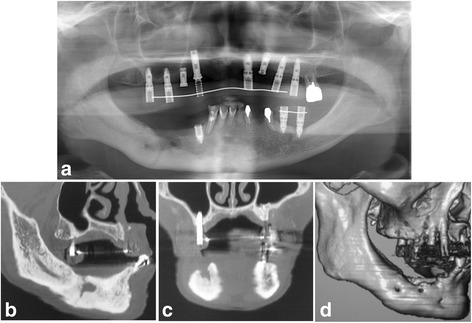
a Panoramic radiograph 16 months after the sequestrectomy. b Sagittal CT view. c Coronal CT view. d 3D CT view
